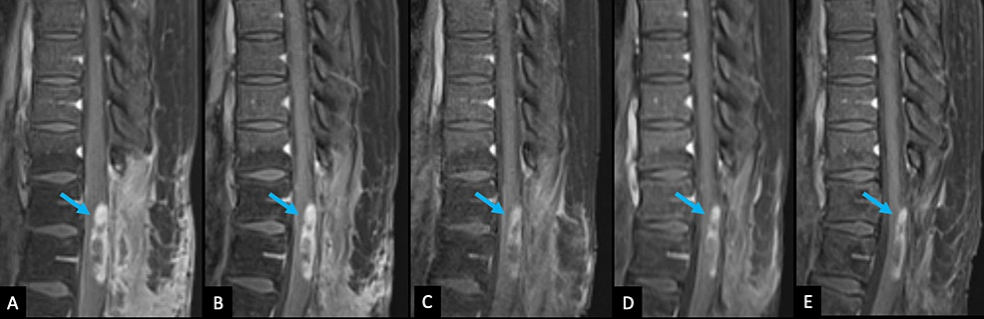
Post-concurrent radiation and chemotherapy MR imaging Serial surveillance MR imaging of the thoracic spine. On the fat-suppressed, post-contrast sagittal T1 weighted image (T1WI), there is progressively decreasing contrast enhancement (blue arrows) associated with the residual tumor at one month (A), four months (B), seven months (C), and 10 months (D). It remains stable over subsequent exams, with the most recent follow-up at 28 months (E).
